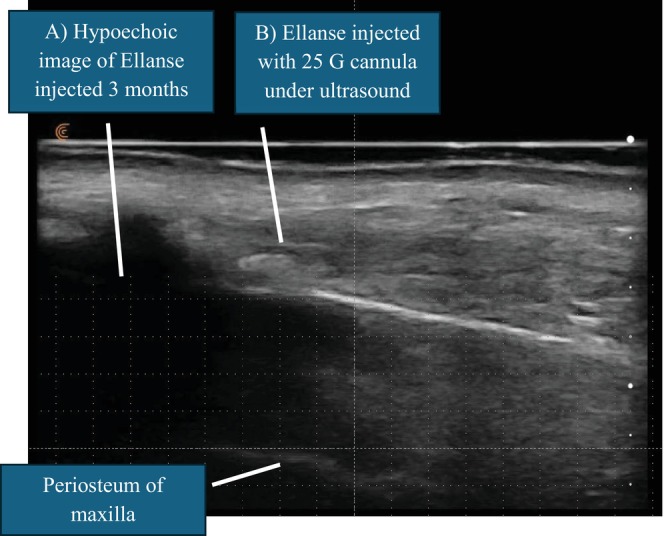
POCUS guided ultrasound‐demonstrating injection of Ellanse in previously treated area. (A) Hypoechoic image indicating previously injected Ellanse 3 months ago. (B) Ellanse injected under ultrasound guidance using a 25 G cannula (Dermasculpt).
